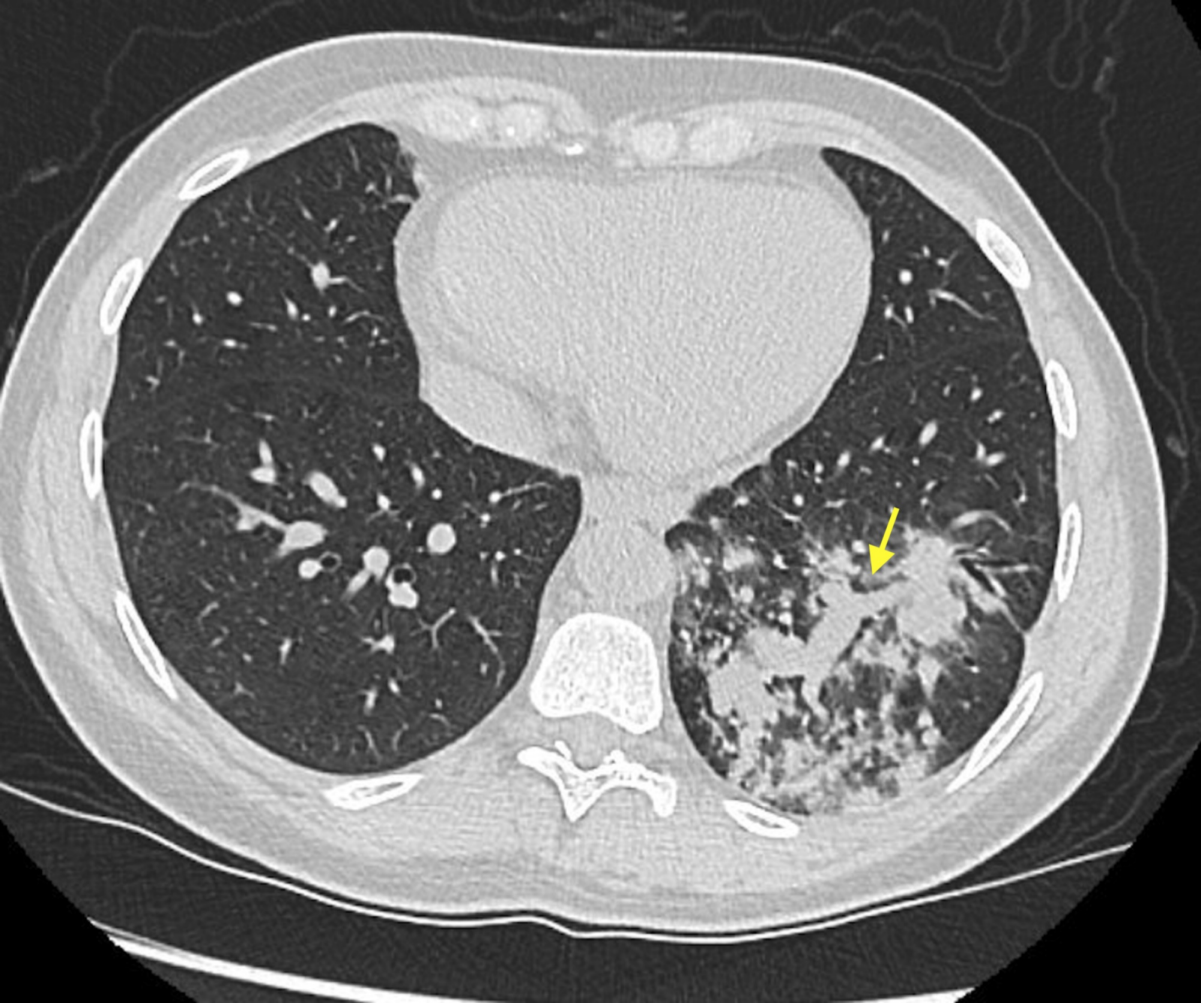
Transverse view of the CT chest showing left lower lobe consolidation (yellow arrow).
